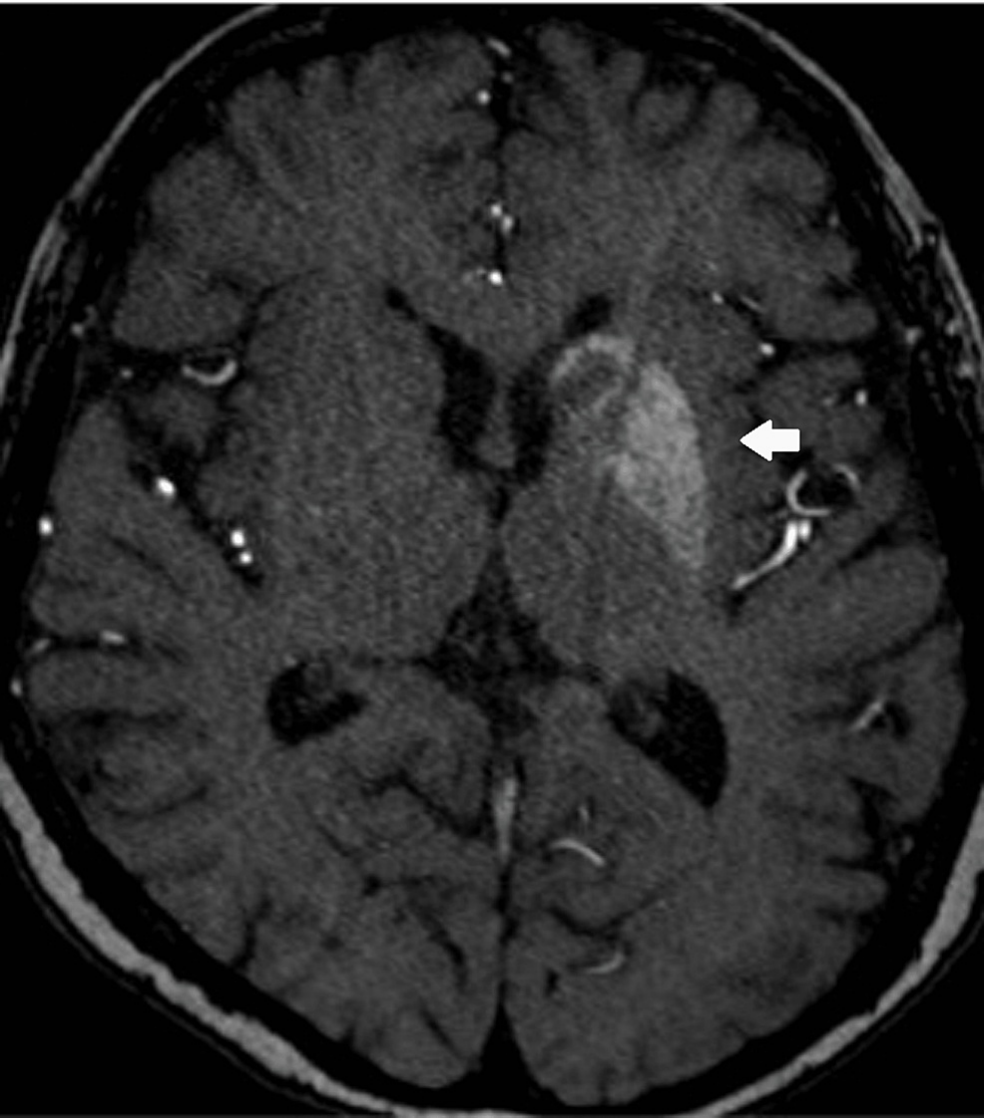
MRI stroke protocol - Time-of-flight magnetic resonance angiography showed persistent T1w hyperintensity of the left basal ganglia
